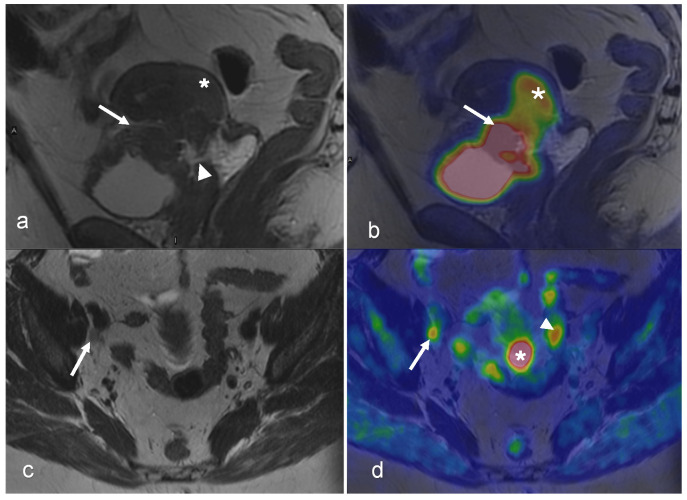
A 54-year-old woman with squamous cell carcinoma of the cervix, FIGO stage IVA. Sagittal T2-weighted MRI (a) shows a 43 mm tumor (star) with indications of invasion into the upper vagina and bladder (arrow) with suspected vesicovaginal fistula (arrowhead). Sagittal-fused PET/MRI (b) shows the FDG uptake of the tumor (star) and bladder wall (arrow). Both axial T2-weighted image (c) and axial-fused PET/MRI (d) demonstrate an 8 mm lymph node with irregular margins and pathologic FDG uptake (arrow in (c,d)). On axial-fused PET/MRI, (d) there is pathologic FDG uptake corresponding to the cervical tumor (star in (d)), which is difficult to distinguish on the axial T2-weighted image. Physiological FDG uptake in the bowel is seen on axial-fused PET/MRI (arrowhead in (d)). (Courtesy of Elisabeth Hedlund, MD, Håkan Ahlström, MD, and Björg Jónsdóttir, MD, PhD, Uppsala University, Uppsala, Sweden).
